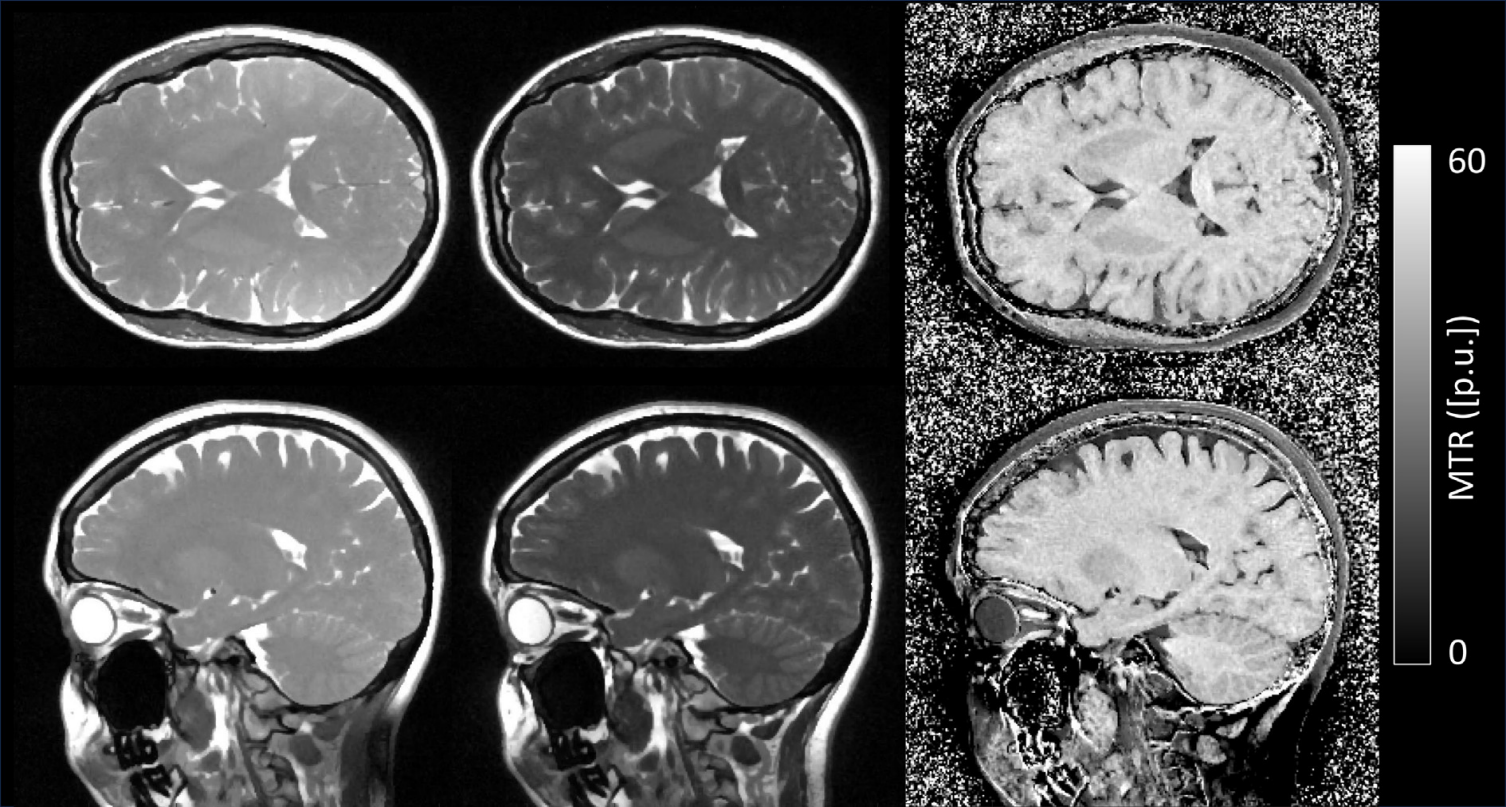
MTR brain imaging using bSTAR. Example slices obtained in volunteer #2 are shown in axial and sagittal orientations for non-MT-weighted (left column) and MT-weighted (middle column) bSTAR MRI with corresponding MTR image (right column).
